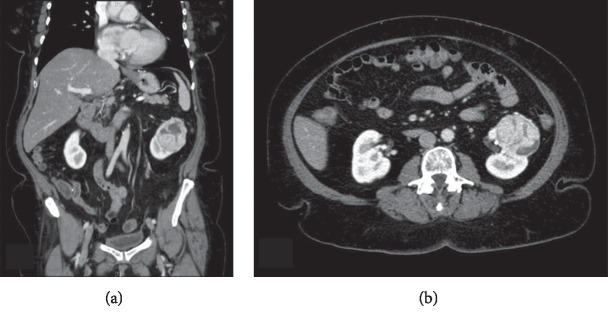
CT A&P demonstrating a 4.9 cm × 5.0 cm × 5.8 cm renal mass. Coronal image (a), axial (b).
